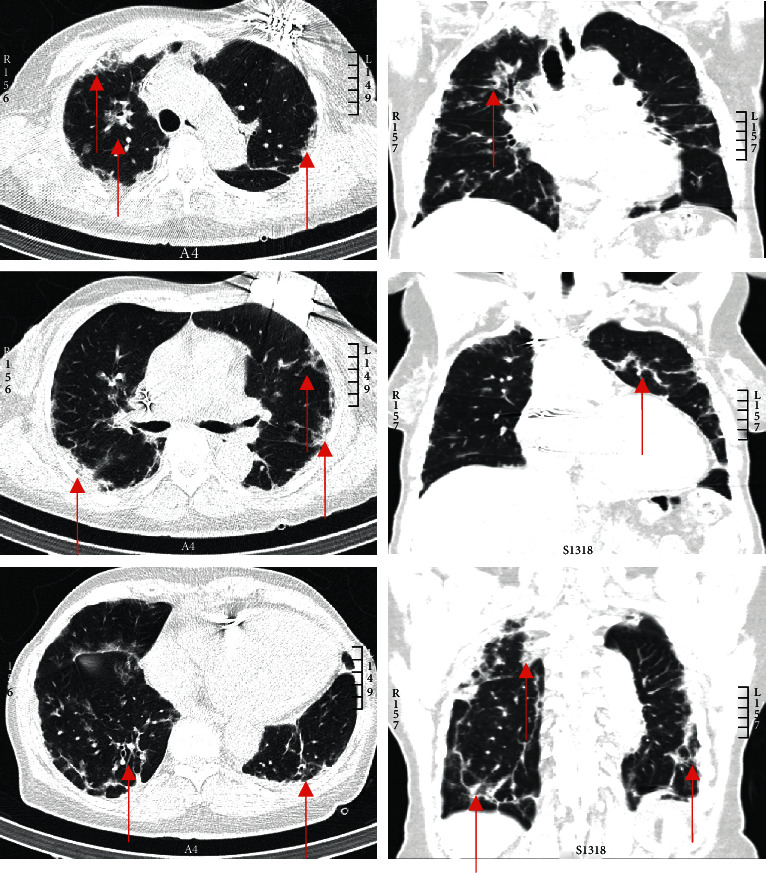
Overview of thoracic CT-scan revealing important COVID-19 disease. The arrows show the bilateral multifocal subpleural ground glass opacities, peribronchovascular infiltrates with partial consolidation, and thin pleural strands in both lower lobes.
